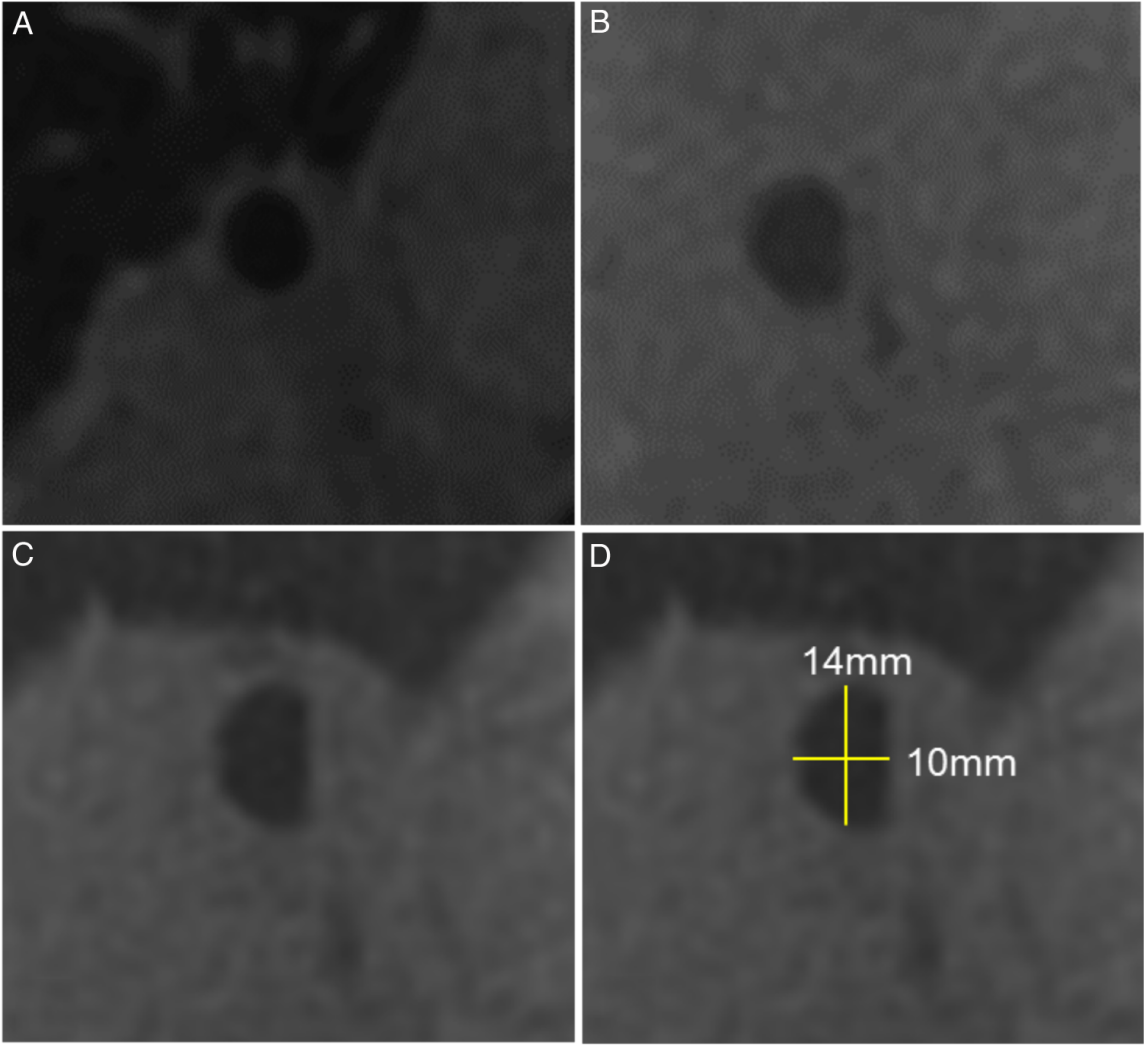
Bronchial diameters by magnetic resonance imaging. Examples of different bronchial shapes obtained from multiplanar reconstructions of the ZTE sequence: (A) round, (B) oval, (C) semilunar, (D) semilunar shape including diameter measurements.
